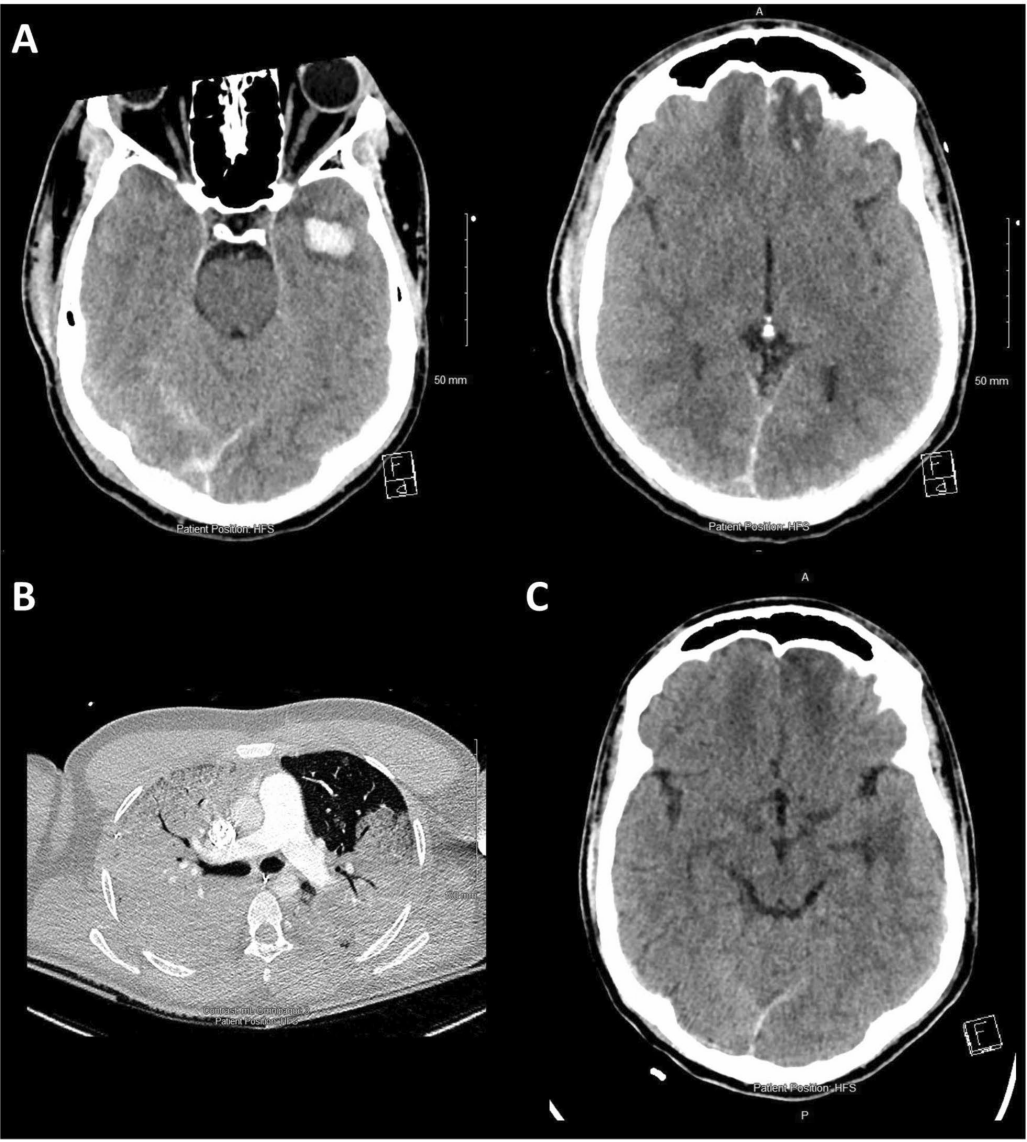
Imaging from case 2. Panel A shows the initial cranial CT scan with a frontotemporal contusion and a traumatic subarachnoid hemorrhage in addition to a parieto-temporal and base skull fractures. Panel B shows the thoracic CT scan displaying the extensive pulmonary consolidations and emboli. Panel C displays the imaging performed at the end of the hospital stay after cessation of extracorporeal membrane oxygenation. The hemorrhage seen on the initial imaging has been absorbed and only residual gliosis can be seen
